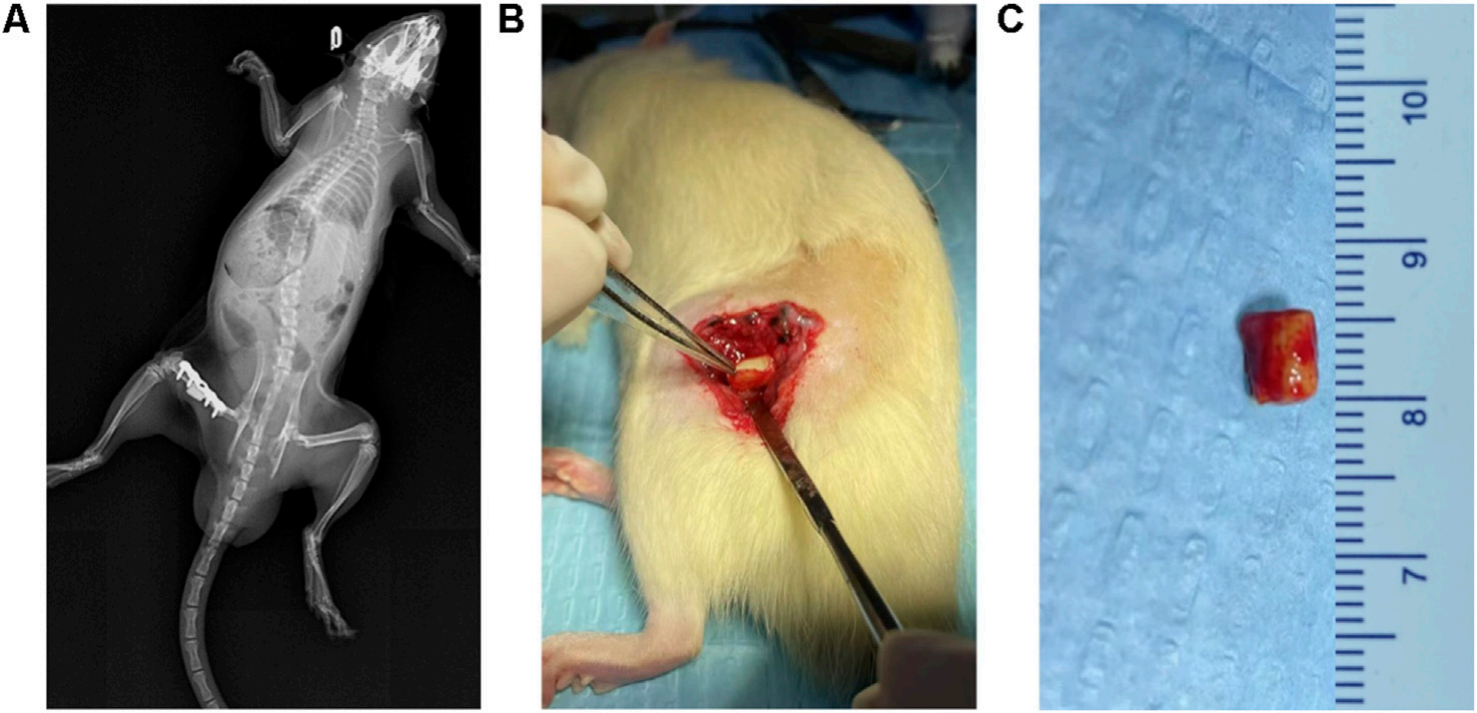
Establishment of IMT models. (A) X-ray images after the first stage of the IMT. The plate was firmly fixed and the PMMA Spacer was not displaced. (B,C) The inducted membrane on the surface of the PMMA Spacer.
